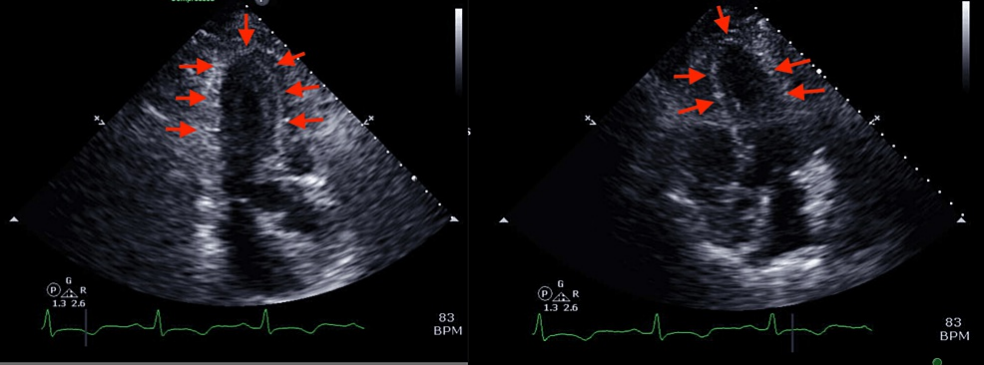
A repeated echocardiogram was performed five days later. Red arrows depict resolved left ventricular apical ballooning seen in initial imaging.
